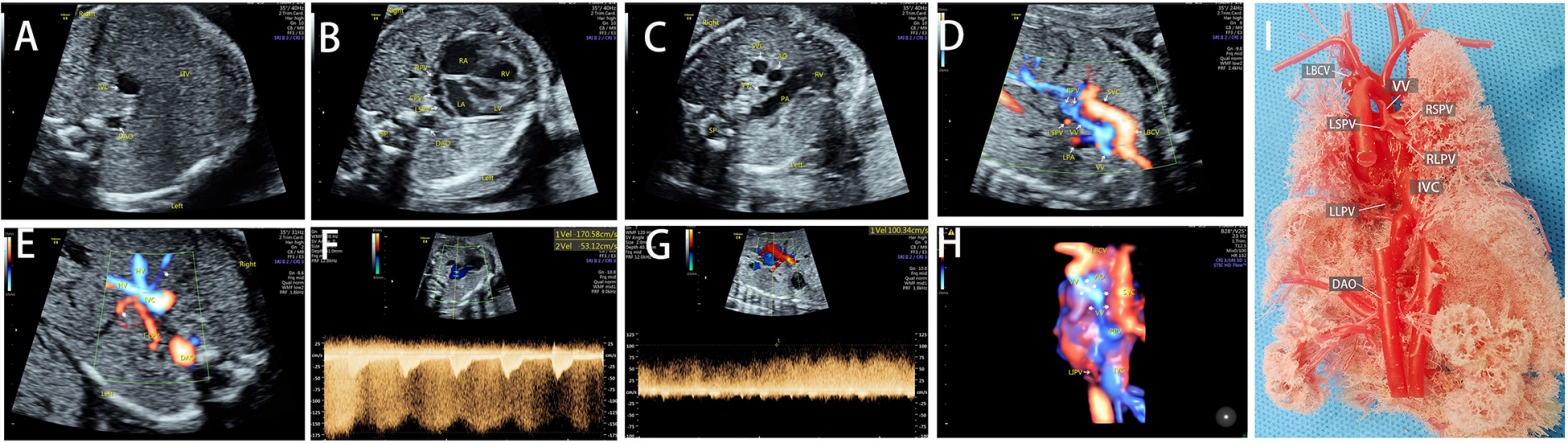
The sonographic features of mixed TAPVC and postnatal anatomic cardiovascular casting findings. (A) A dilated IVC in transabdominal section. (B) The ventricles were asymmetrical and the CPV was located behind the LA. (C) The VV appeared as an extra vessel behind the aorta. (D) The color Doppler showed the drainage of the RSPV, RLPV, and the LSPV into the LBCV via VV. (E) The LLPV drained into IVC. (F,G) Obstruction was confirmed in both the supracardiac and infracardiac pathways. (H) The entire drainage route of all PVs. (I): The postnatal casting of the TAPVC heart showed the RSPV, RLPV, and LSPV drainage into LBCV via the VV, and the LLPV drainage into the IVC. TAPVC, total anomalous pulmonary venous connection; IVC, inferior vena cava; CPV, common pulmonary vein; LA, left atrium; VV, vertical vein; RSPV, right superior pulmonary vein; RLPV, right low pulmonary vein; LSPV, left superior pulmonary vein; LBCV, left brachiocephalic vein; LLPV, left low pulmonary vein.
